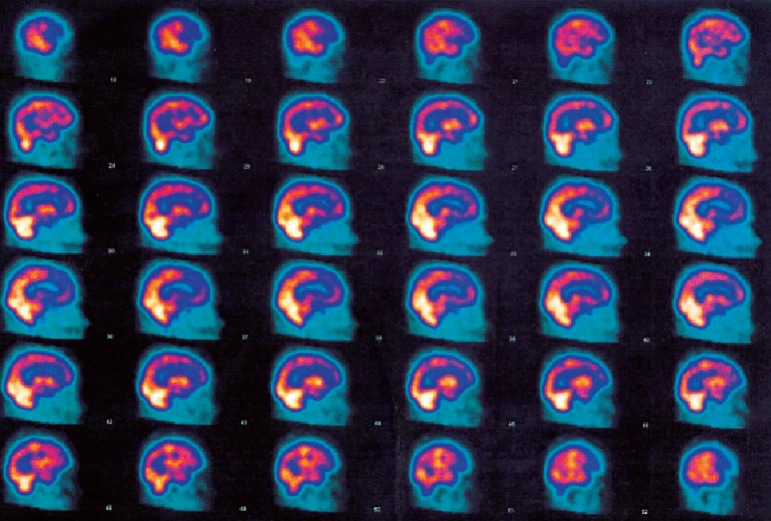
SPECT with Frontal lobes hypoperfusion.
